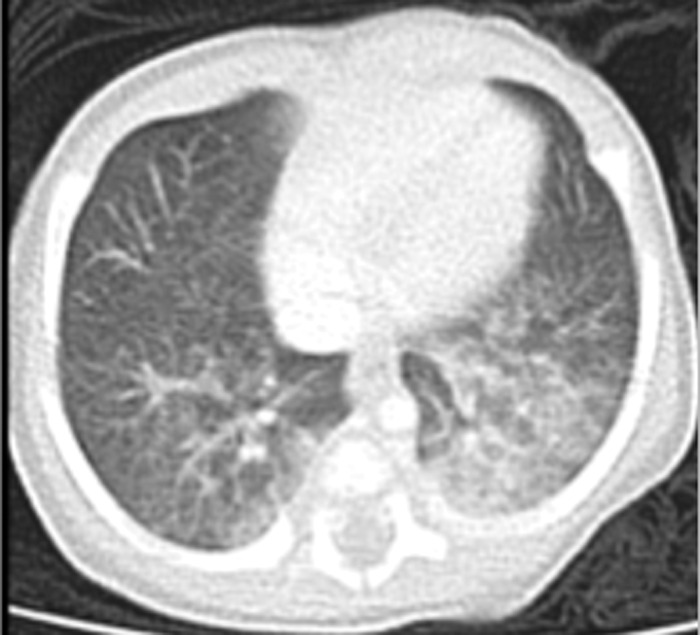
Computed tomography of the chest showed patchy bilateral pulmonary ground glass abnormalities in the upper and lower lobes.
